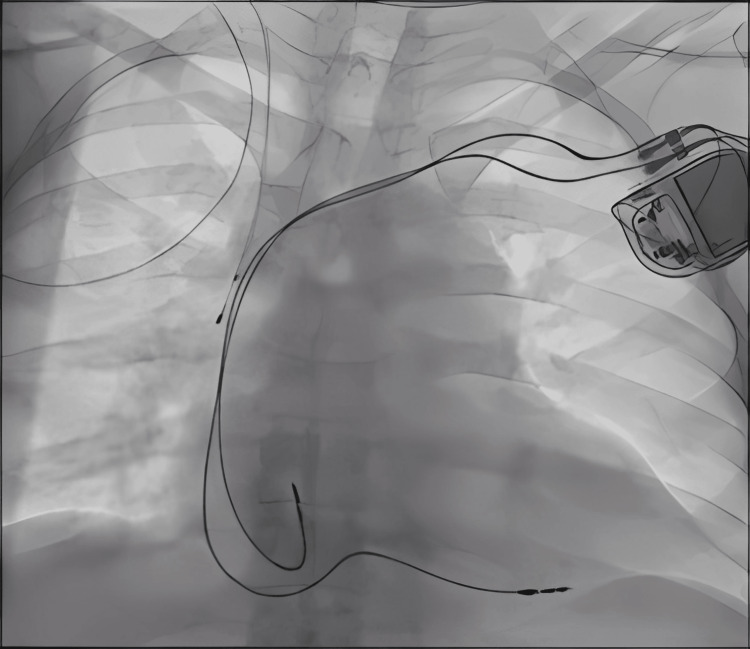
Post-permanent pacemaker insertion chest radiograph confirming proper lead placement
